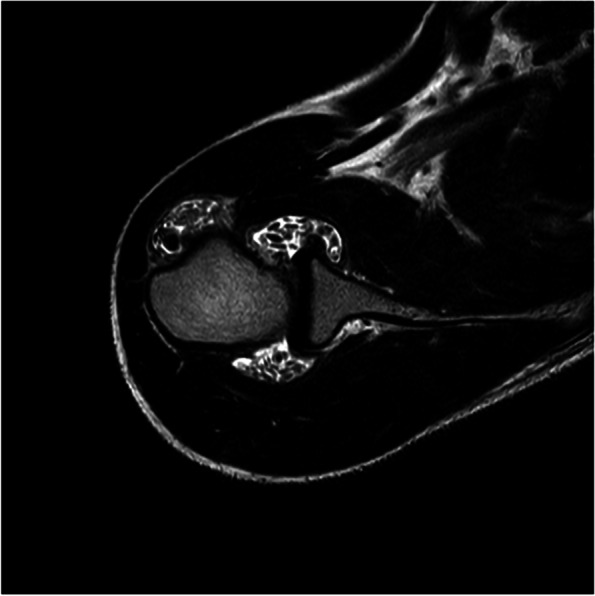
T2-weighted MRI shows a pronounced effusion of the shoulder joint, especially the subacromial bursa and axillary recess. Multiple hypointense lesions are present within the articular space
